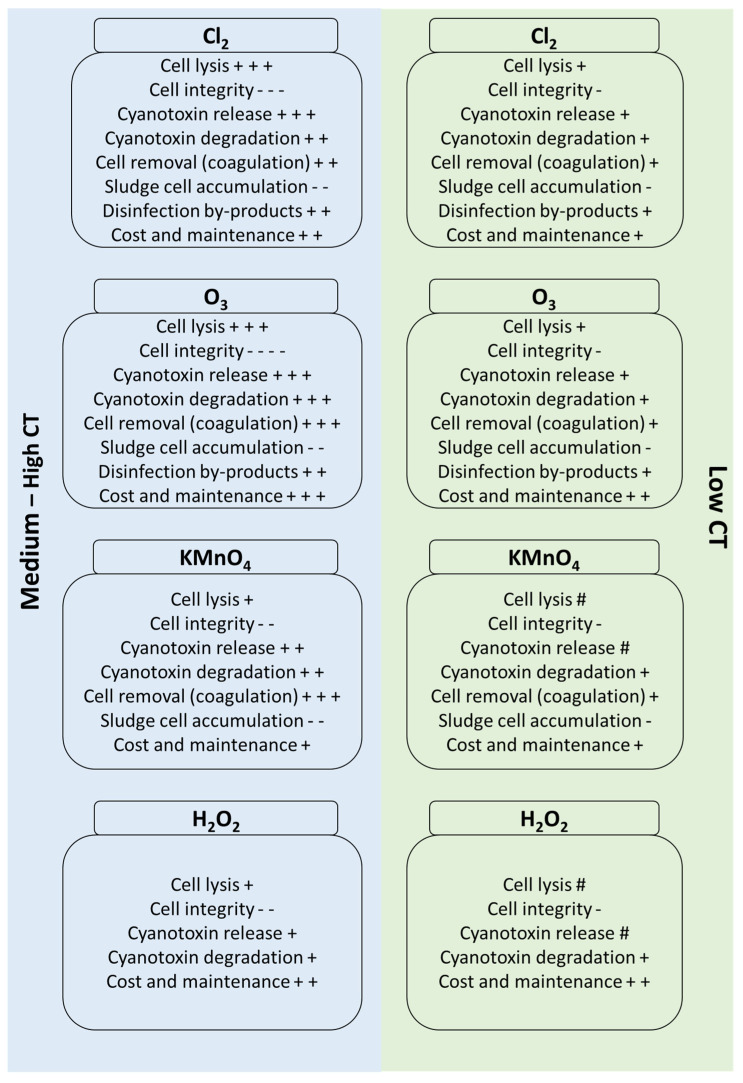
Summary of pre-oxidation (low and medium-high CT) impact on cyanobacteria/cyanotoxins and downflow processes (#: very low impact, +: increase, and -: decrease). Low CT for Cl_2_ = CT < 4 mg min/L, low CT for O_3_ = CT < 1 mg min/L, low CT for KMnO_4_ = CT < 50 mg min/L, and low CT for H_2_O_2_ = CT < 50 mg h/L.
